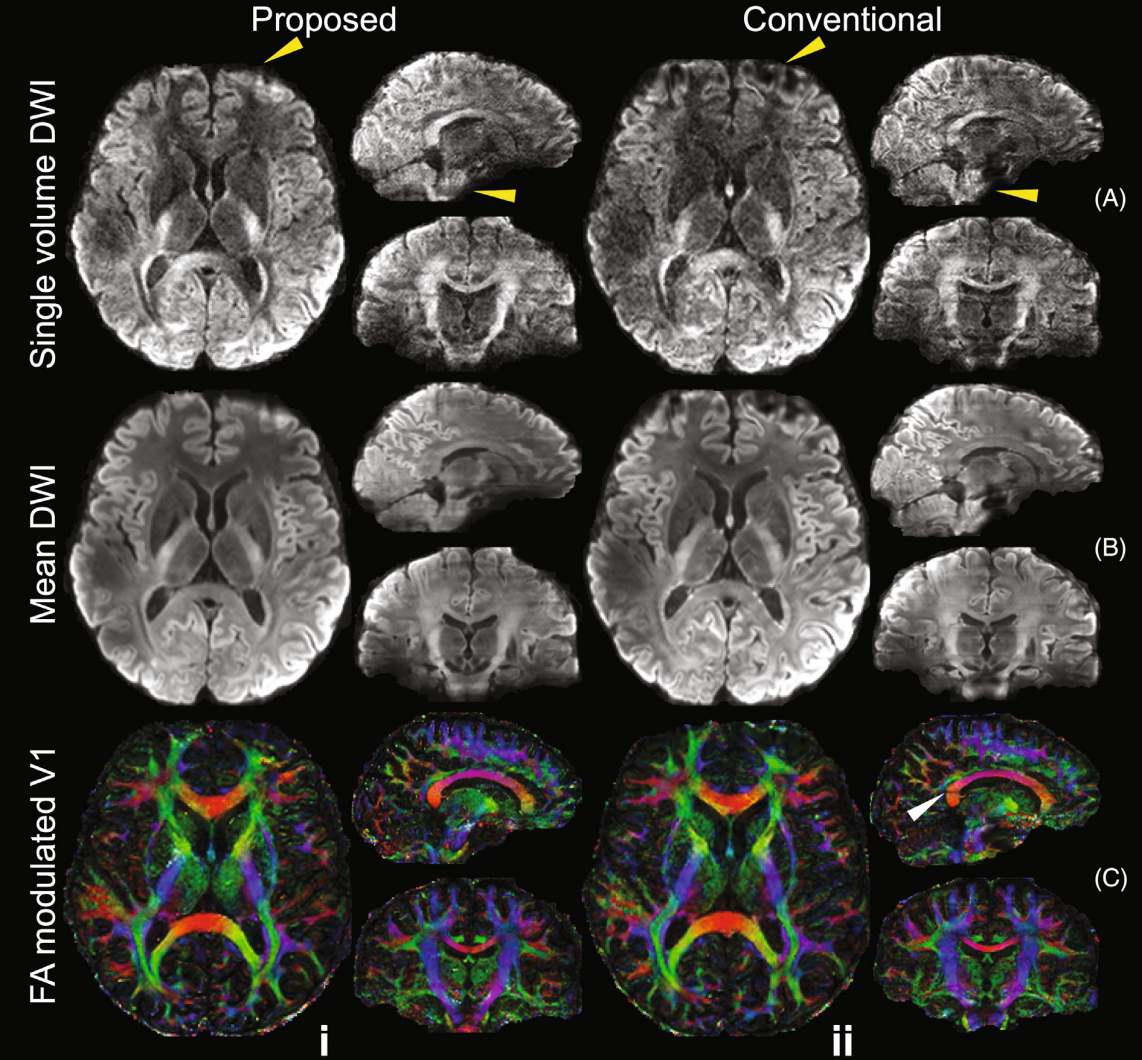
High‐resolution whole‐brain diffusion images. The single volume diffusion‐weighted images (DWI) along the diffusion direction (−0.10, 0.88, 0.47) (A), the mean DWI (B), and the fractional anisotropy (FA) modulated primary eigenvector (V1) (C) of diffusion data acquired using joint blip‐reversed with CAIPI‐PF sampling (Proposed, i) and conventional 3D multi‐slab sampling using only blip‐up phase encoding (Conventional, ii) at 1.05 mm isotropic resolution from the same subject are displayed. The diffusion data are co‐registered to the same anatomical data (0.86 mm isotropic resolution) for comparison.
